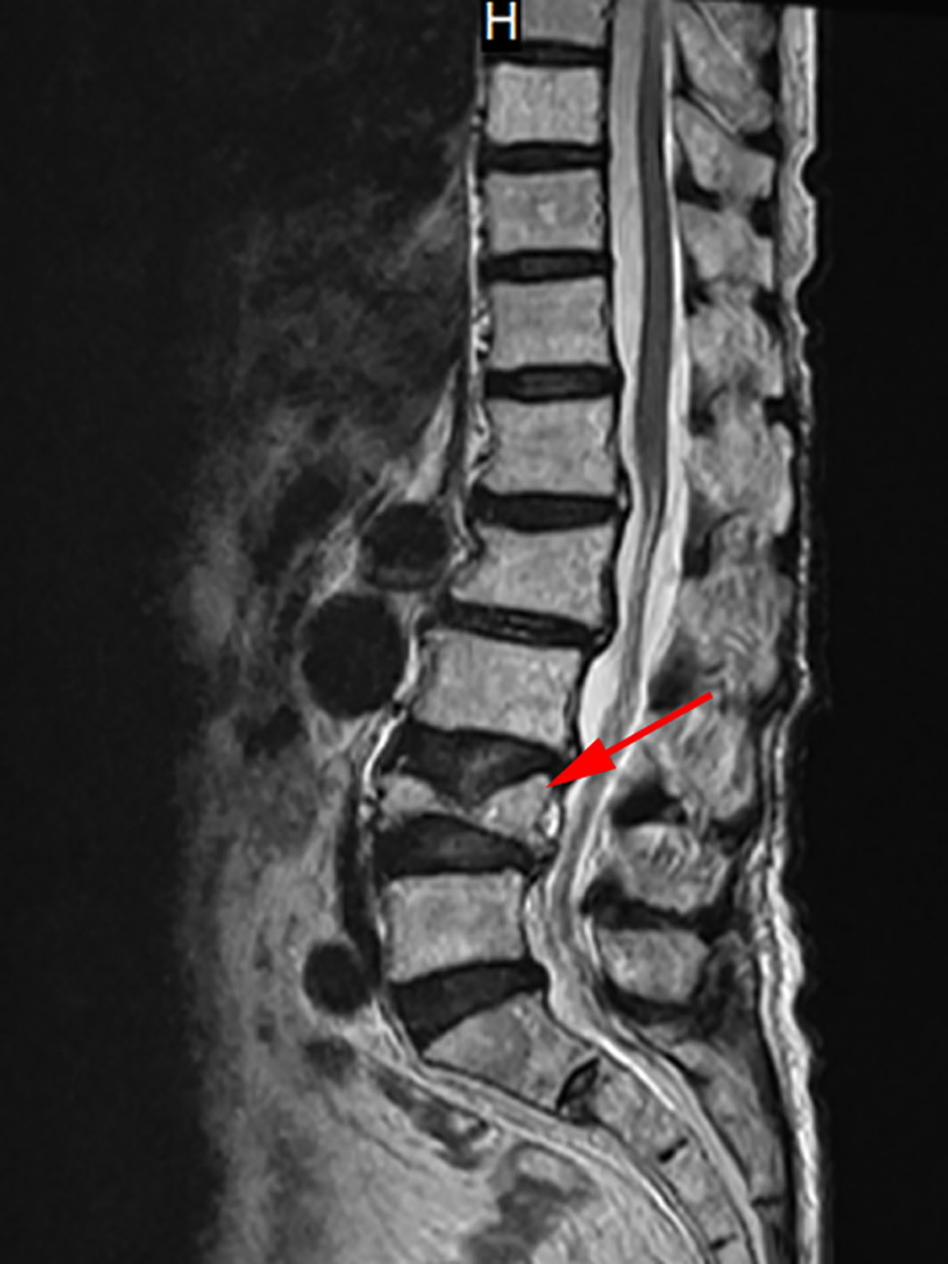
MRI of the lumbar vertebra. This image shows the MRI of the patient’s lumbar vertebra. The red arrow marks the 4th lumbar vertebra with a compression fracture.
